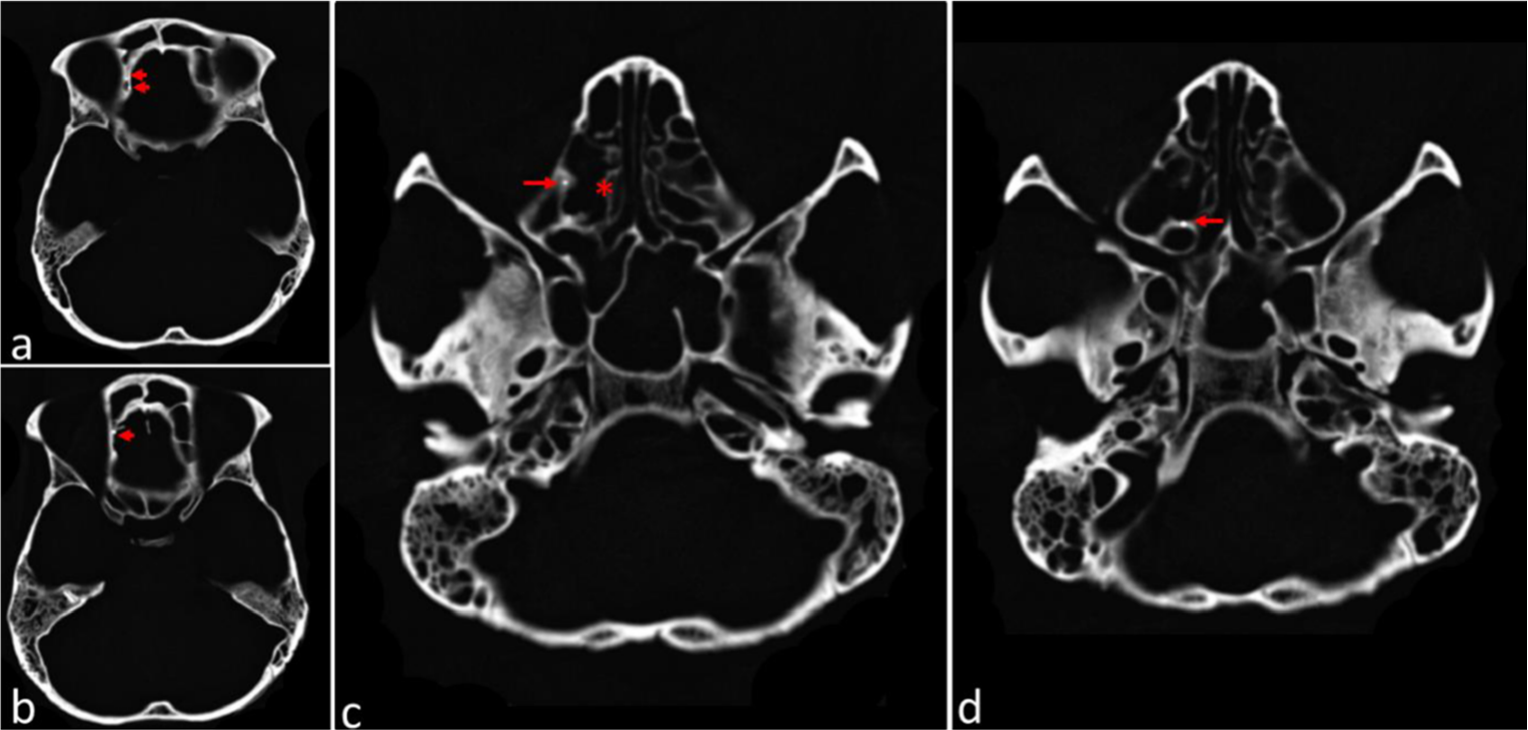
Axial CT images at the level of the ethmoidal labyrinth and orbital cavity. 4a: the right orbital blade of ethmoid bone had an area with images of high radiological density (red arrows). 4b: at the ethmoidal bone, behind the right side of the frontal sinus, there was an image of high radiological density (red arrow). 4c and 4d: the bone framework of the right ethmoidal cells had an area with an image of high radiological density (red arrow). The ethmoidal cells on the right (red asterisk), compared to the left, had areas of bone loss, which indicates the path and damage caused by the firearm projectile
